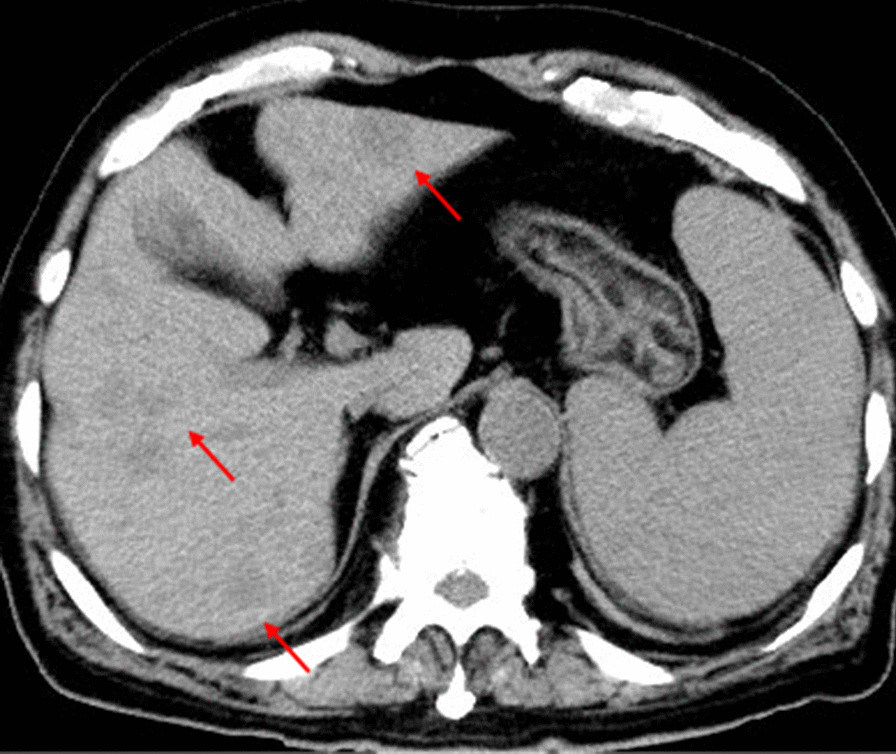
Computed tomography findings. Multiple hypoattenuating hepatic nodules without a dominant mass (red arrows). Splenomegaly was also seen
